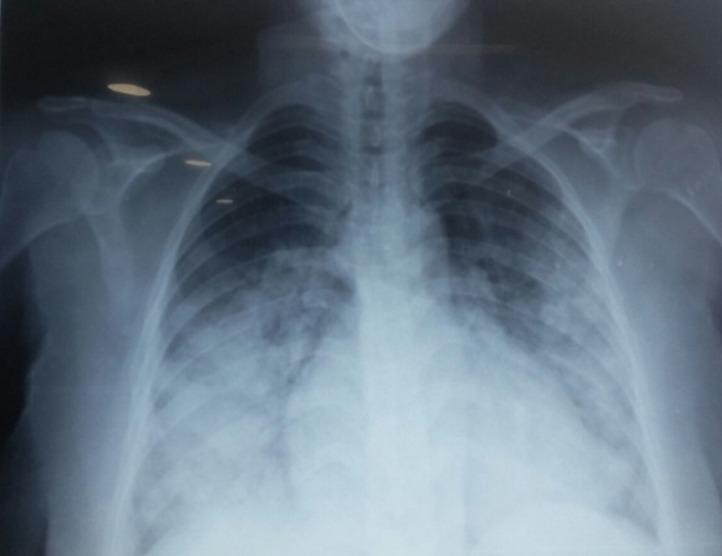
Radiographie pulmonaire de face: surcharge péri-hilaire en faveur d’un OAP
